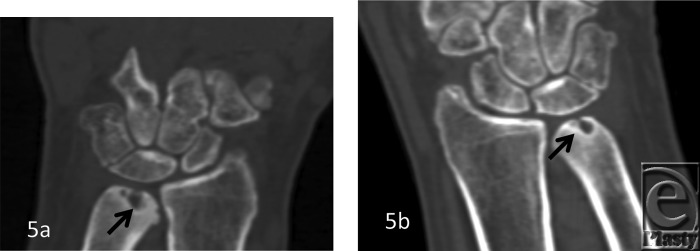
A 45-year-old man with diffuse wrist pain. Outside plain films not available. 2D reconstructions from the kinematic 4D CT volume scan show ulnar sided carpal translocation bilaterally. Also note subchondral cystic changes of ulna bilaterally (*arrows*) suggesting dynamic ulnocarpal abutment.
